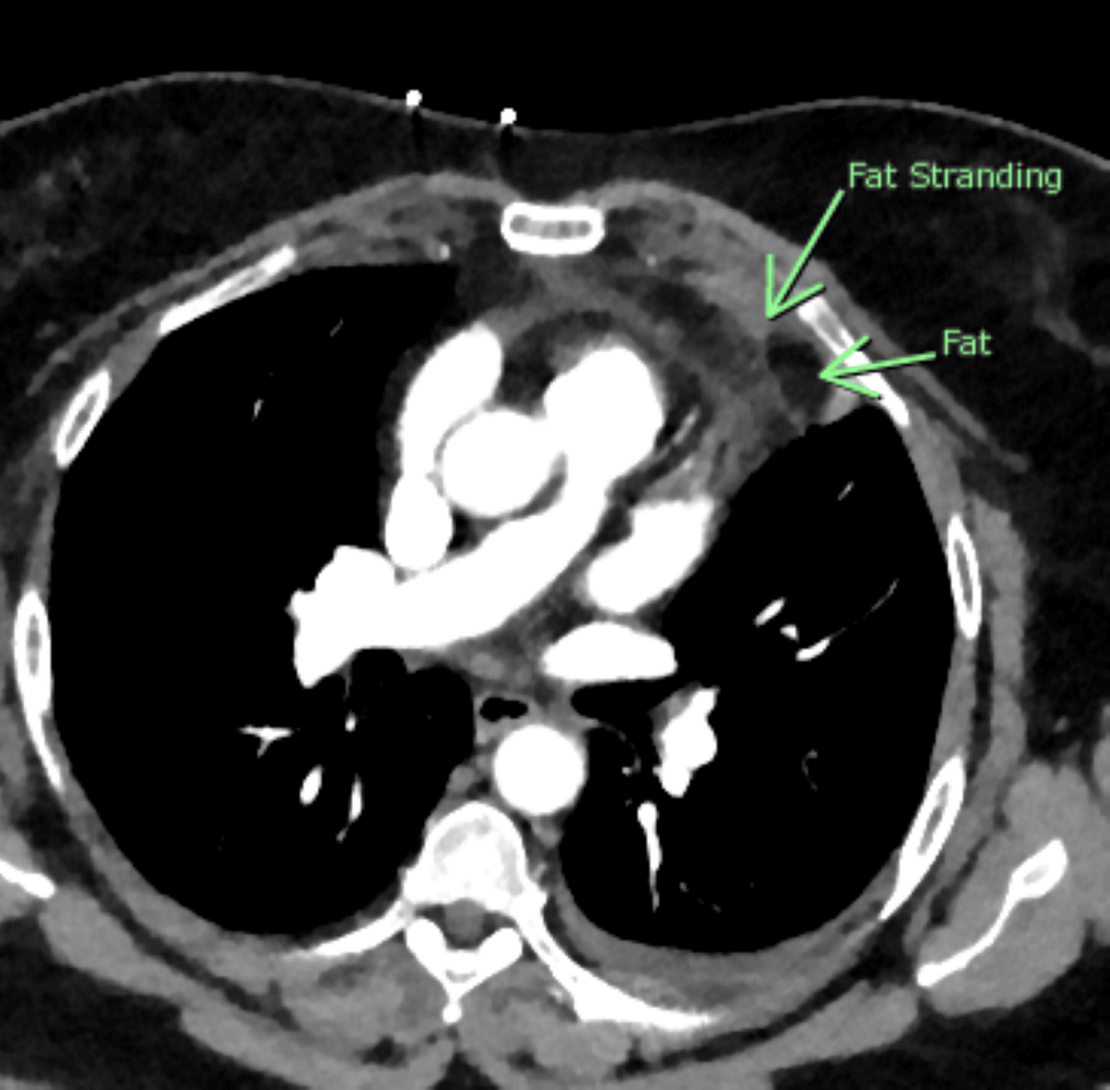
Axial view of CT showing a 4.2 cm x 1.8 cm x 3 cm lesion in the left epicardial space, surrounded by an area of adjacent fat stranding suggestive of central necrosis.
